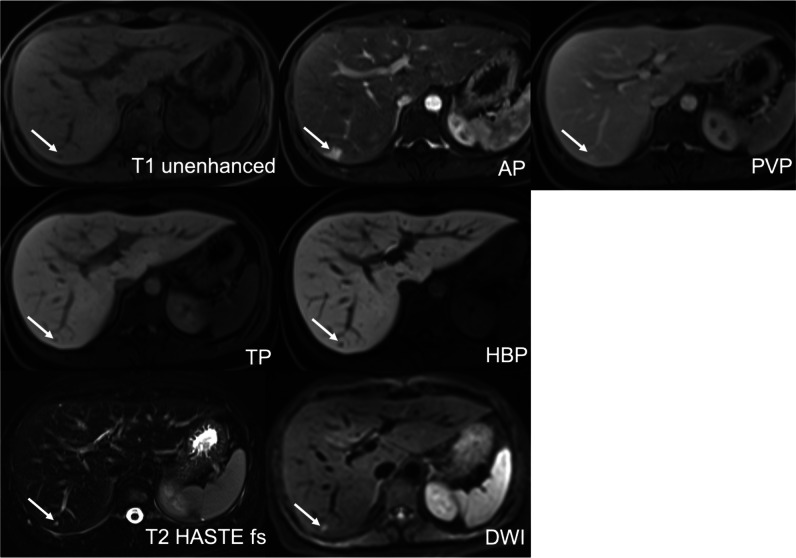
No AP artifacts, score 1, optimal timing, excellent lesion visibility, score 5: This 31-year-old woman was sent for MRI after an inconclusive ultrasound regarding a small focal liver lesion. A tiny T1-hypointense, markedly T2- and DWI-hyperintense lesion is seen in segment VI. On dynamic imaging, it shows strong corona enhancement in the AP, retained GA in the PVP, and pseudo-washout in the TP and HBP. There was no restriction on the ADC (not shown). The lesion is consistent with a flash-filling hemangioma
